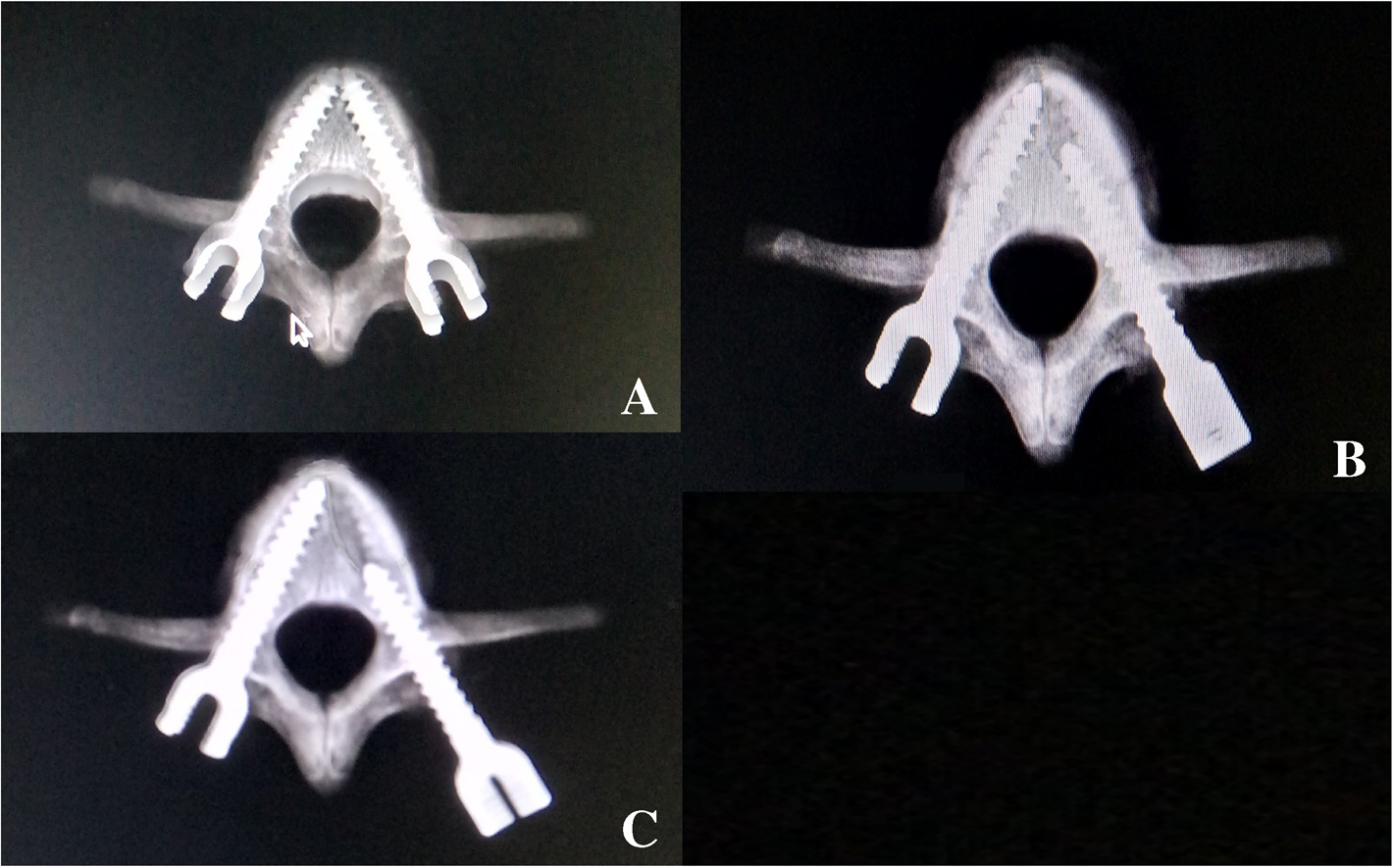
Radiographs identifying the depth of insertion. a Experimental screw was removed after being completely inserted in one pedicle and was then reinserted completely. b Experimental screw was removed after 80% of the total trajectory was reached and then reinserted completely. c Experimental screw was removed after 60% of the total trajectory was reached and then reinserted completely
